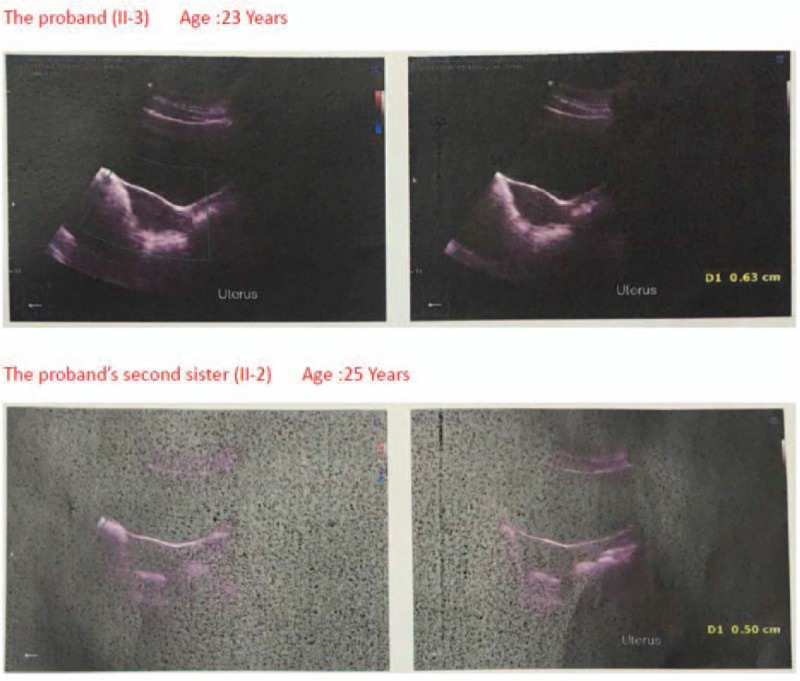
Ultrasound image of patients: proband and her second eldest sister both appeared small uterus.
